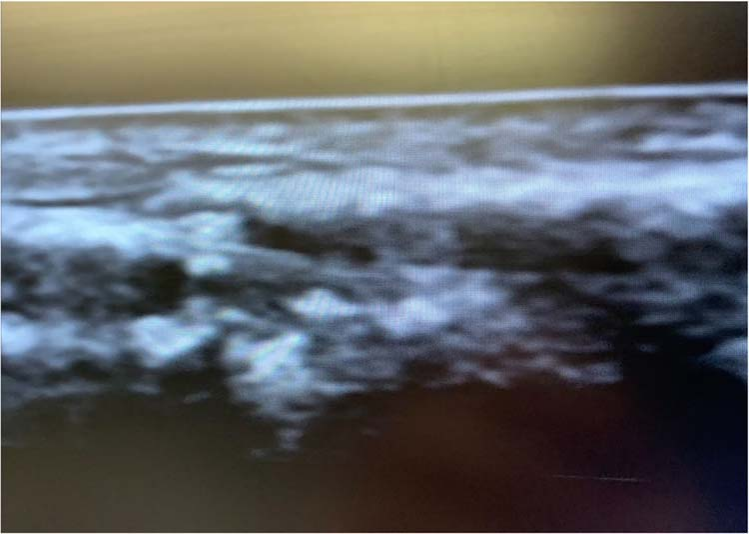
Non-color doppler showing retained arterial line.
